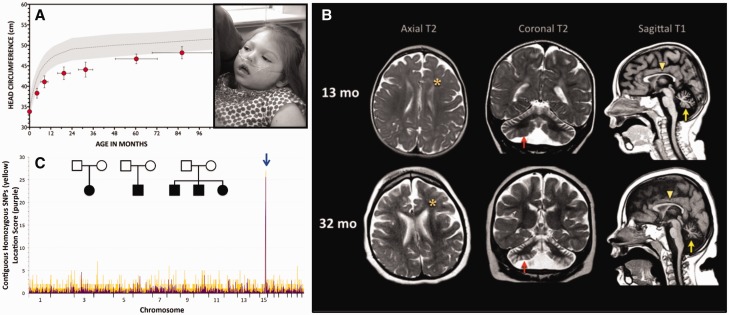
Nephrocerebellar syndrome. (A) Postnatal brain growth is slow in children with NCS (grey-shaded area = normal head circumference for age, mean ± 2 SD; red circles = NCS head circumference, mean ± SD). *Inset*: Children with NCS have no distinctive dysmorphic features, but the forehead characteristically recedes, reflecting relative hypoplasia of the cerebral cortex; post-mortem brain weights are only 50–60% of normal (photos used with parental permission). (B) MRI at 1.5 T (left to right: axial T_2_, coronal T_2_, sagittal T_1_) shows underdeveloped frontal lobes (asterisks), thin corpus callosum (yellow arrowheads), and severe atrophy of the cerebellar hemispheres (red arrows) and vermis (yellow arrows). Comparable images of the same NCS child, taken at 13 months (*upper panel*) and 32 months (*lower panel*), reveal progressive degeneration of cerebellar tissue (arrows). (C) Initial SNP genotyping of five children (*inset*) mapped recessive NCS to an 8.26 Mb autozygous block of DNA on chromosome 15 (blue arrow). After the initial mapping, we identified nine additional patients that allowed us to narrow the shared homozygous interval to 3.96 Mb. Yellow signal indicates the number of contiguous SNPs shared among NCS individuals. For each block of shared homozygous SNPs, purple signal shows the cumulative two-point LOD score (‘location score’), a relative probability, based on population allele frequencies, that the disease gene resides in the homozygous block. Subsequent genotyping of all 27 NCS individuals refined the shared locus to 700 kb, which contained the novel *WDR73* c.888delT mutation homozygous in all affected children.
